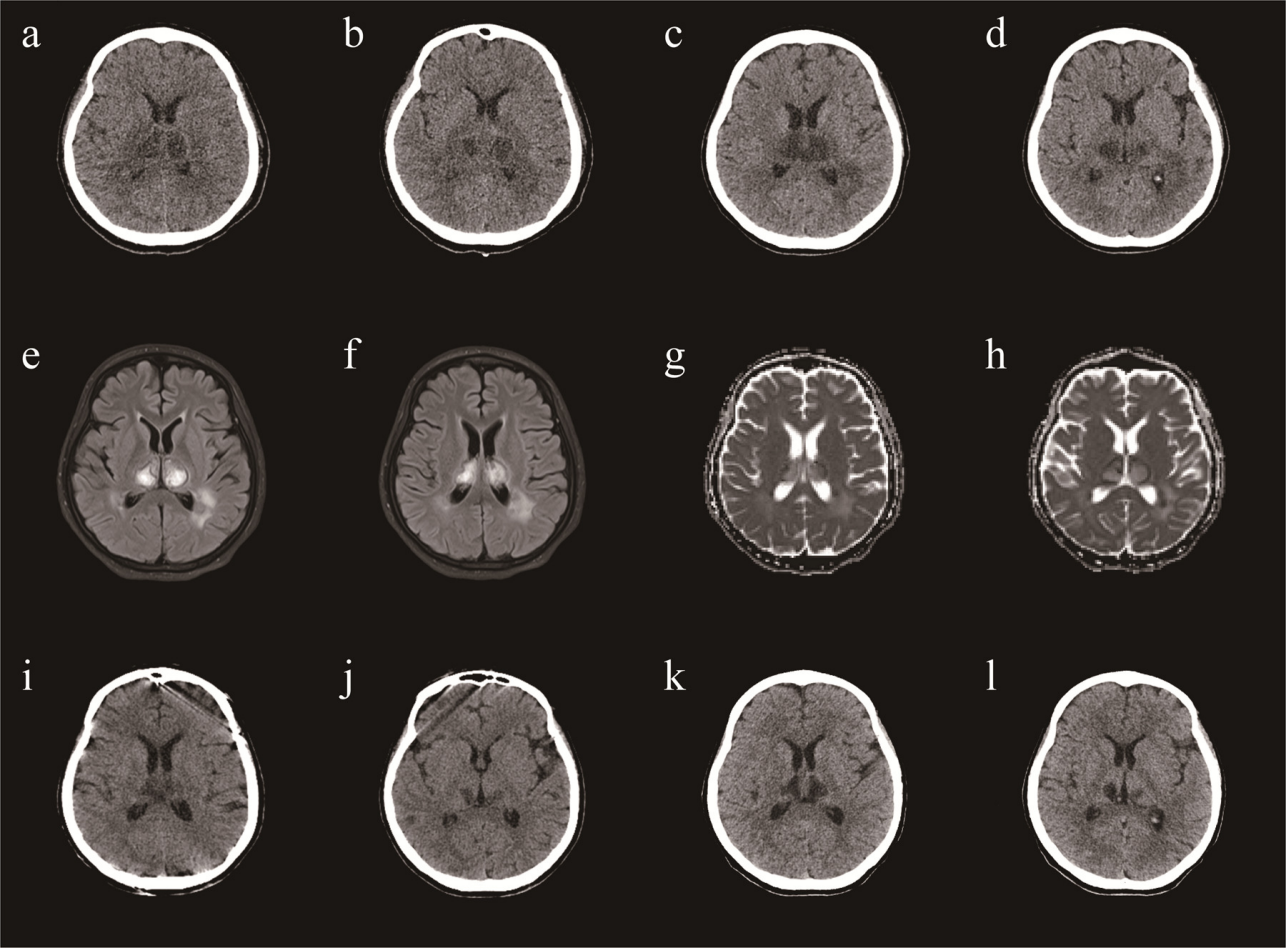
A brain CT (a,b) demonstrating symmetric lesions involving thalami, striatum and brain stem, consistent with acute necrotizing encephalopathy in day 12. A repeat CT revealed that the lesions in the head had reduced (c,d) in day 20. A repeat CT revealed that the lesions in the head had reduced (e,f) in day 38. One month after discharge, a repeat CT revealed that the lesions in the head had reduced (g,h). MRI (i,j) demonstrating symmetric lesions involving thalami, striatum and brain stem, consistent with acute necrotizing encephalopathy in day 22.
